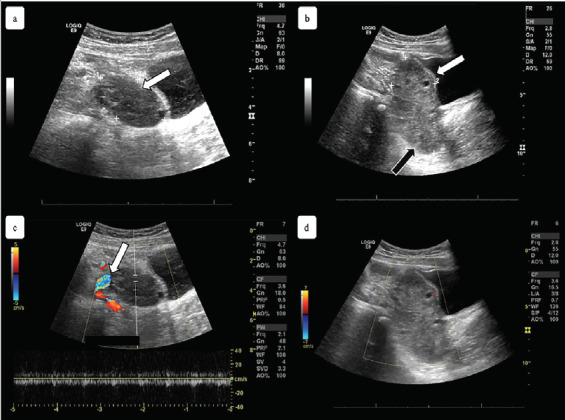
a) Sagittal view of the patient’s left ovary on transabdominal pelvic ultrasound, measuring 4.4 × 2.4 × 2.0 centimeters (cm), indicating a slightly enlarged but otherwise normal ovary (white arrow); b) sagittal view of the patient’s right ovary with measurements of 8.7 × 1.3 × 6.1 cm, demonstrating an abnormally enlarged ovary (white arrow) with a heterogenous mass (black arrow); c) sagittal view of the patient’s left ovary with normal color flow Doppler (white arrow); d) sagittal view of the patient’s right ovary demonstrating lack of blood flow on color flow Doppler.
